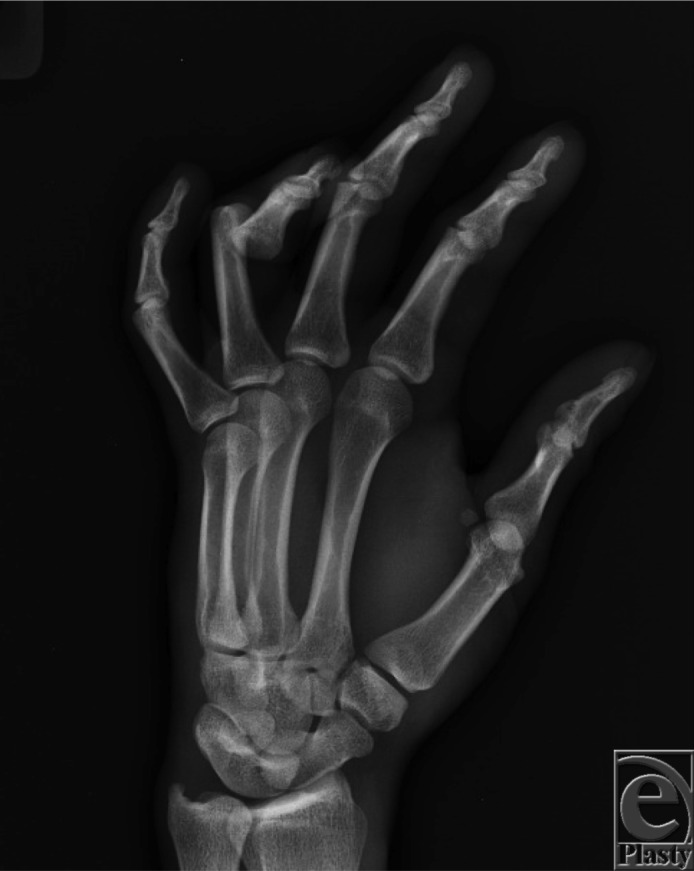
Radiograph from initial presentation demonstrating volar proximal interphalangeal joint dislocation of the fourth digit.
